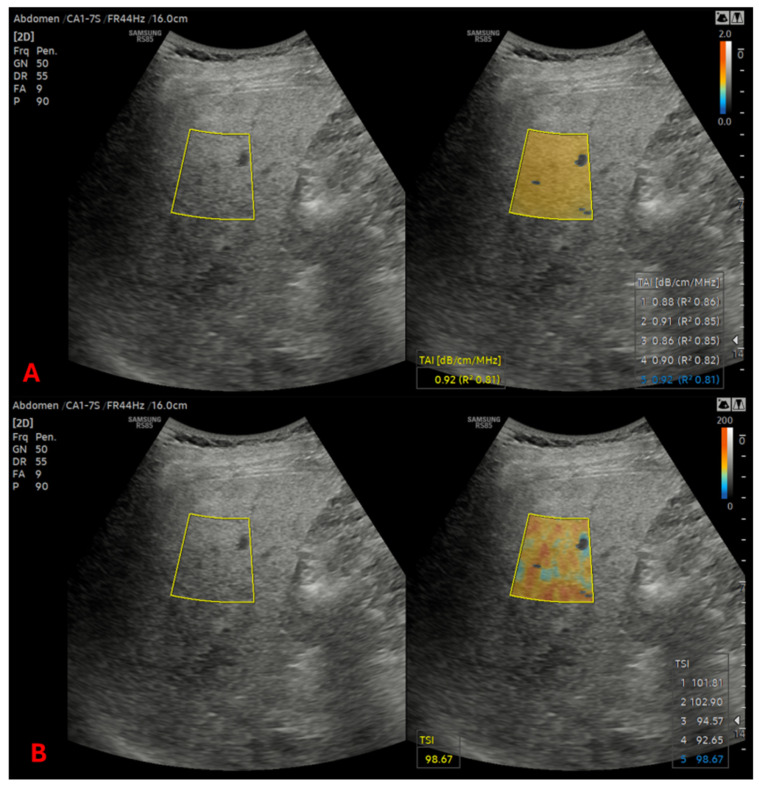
Quantitative ultrasound measurement of liver fat. Tissue attenuation imaging (A) and tissue scatter distribution imaging (B) of participants with moderate liver fat content of more than 5% less than 15%. Shear wave elastography was applied which ruled out significant fibrosis.
